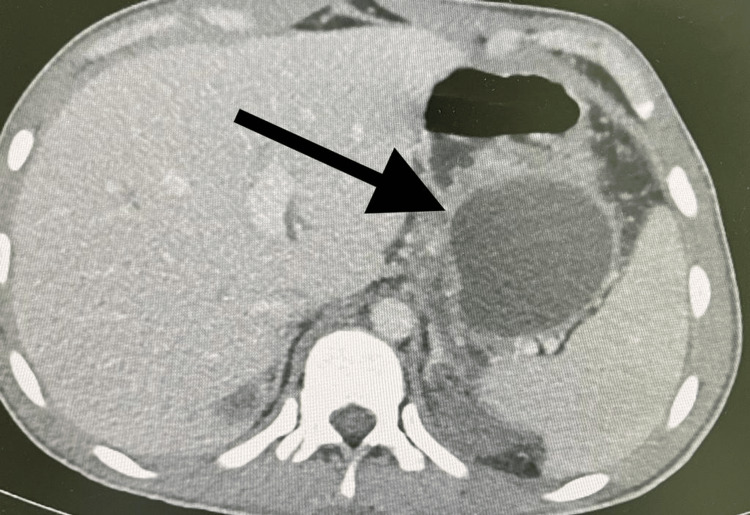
CT scan with contrast of the abdomen and pelvis, axial view showing pancreatic pseudocyst.
